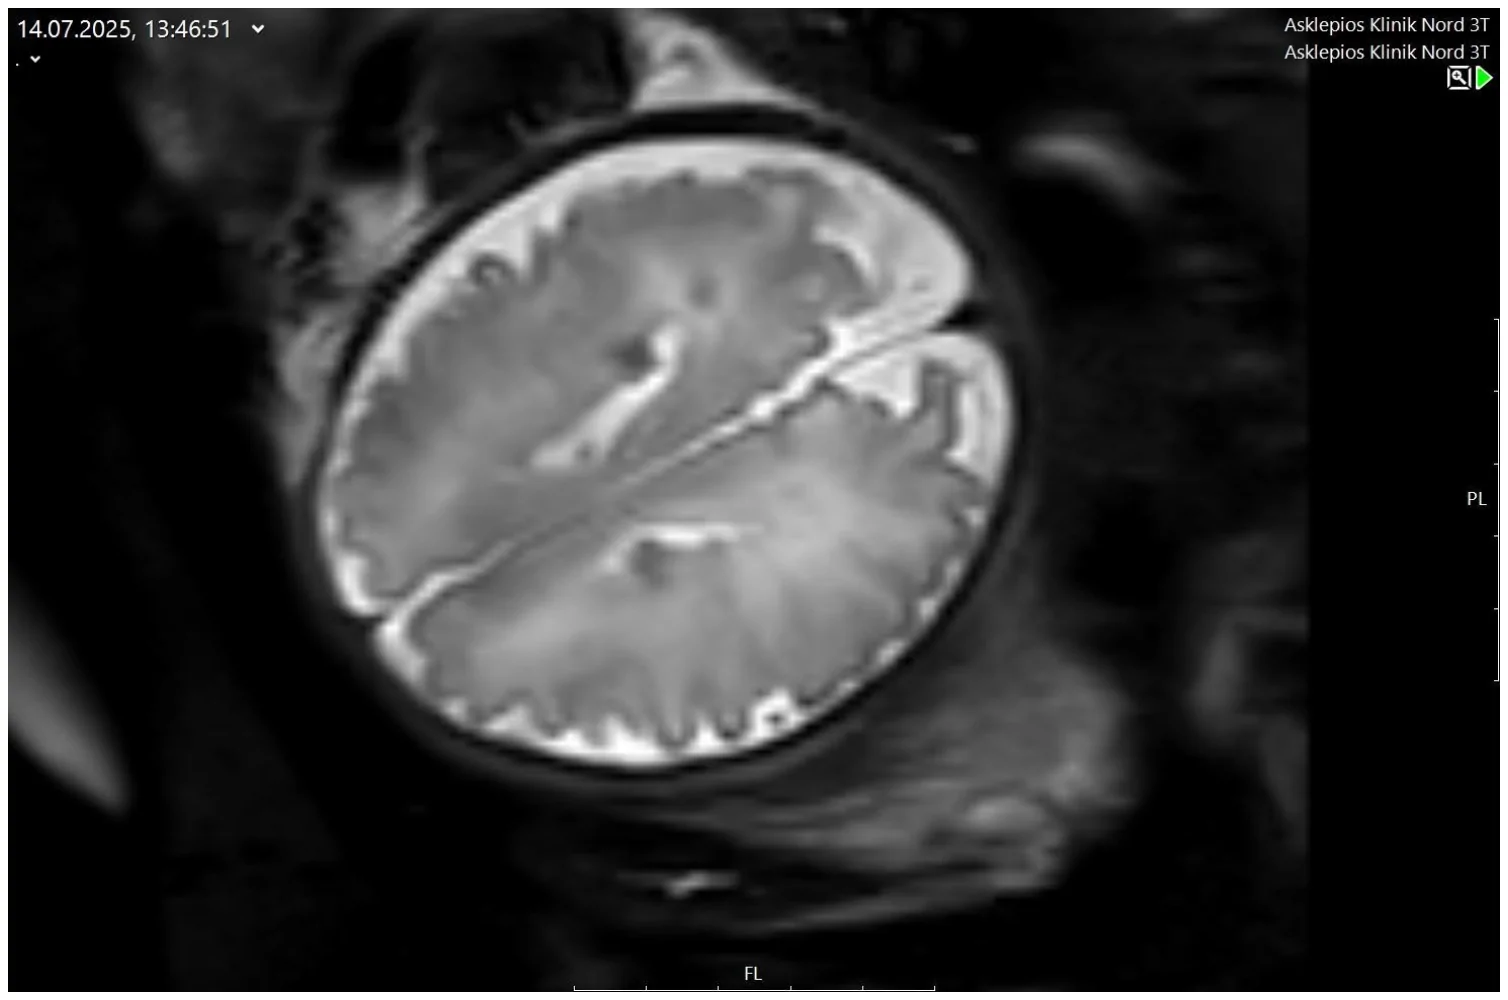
Fetal magnetic resonance imaging demonstrating bilateral T2-hypointense subependymal lesions, compatible with calcified subependymal nodules in the setting of tuberous sclerosis complex.
